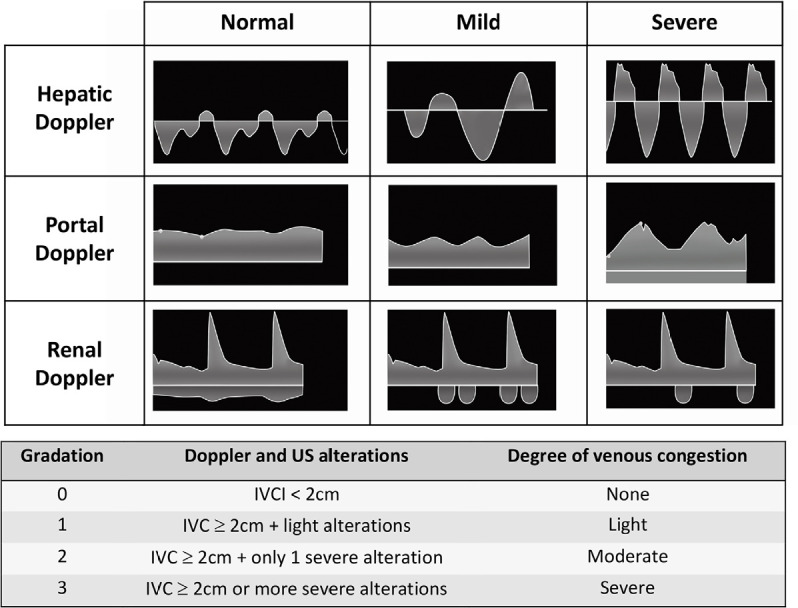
Pulsed Doppler ultrasound pattern of the hepatic, portal and renal
